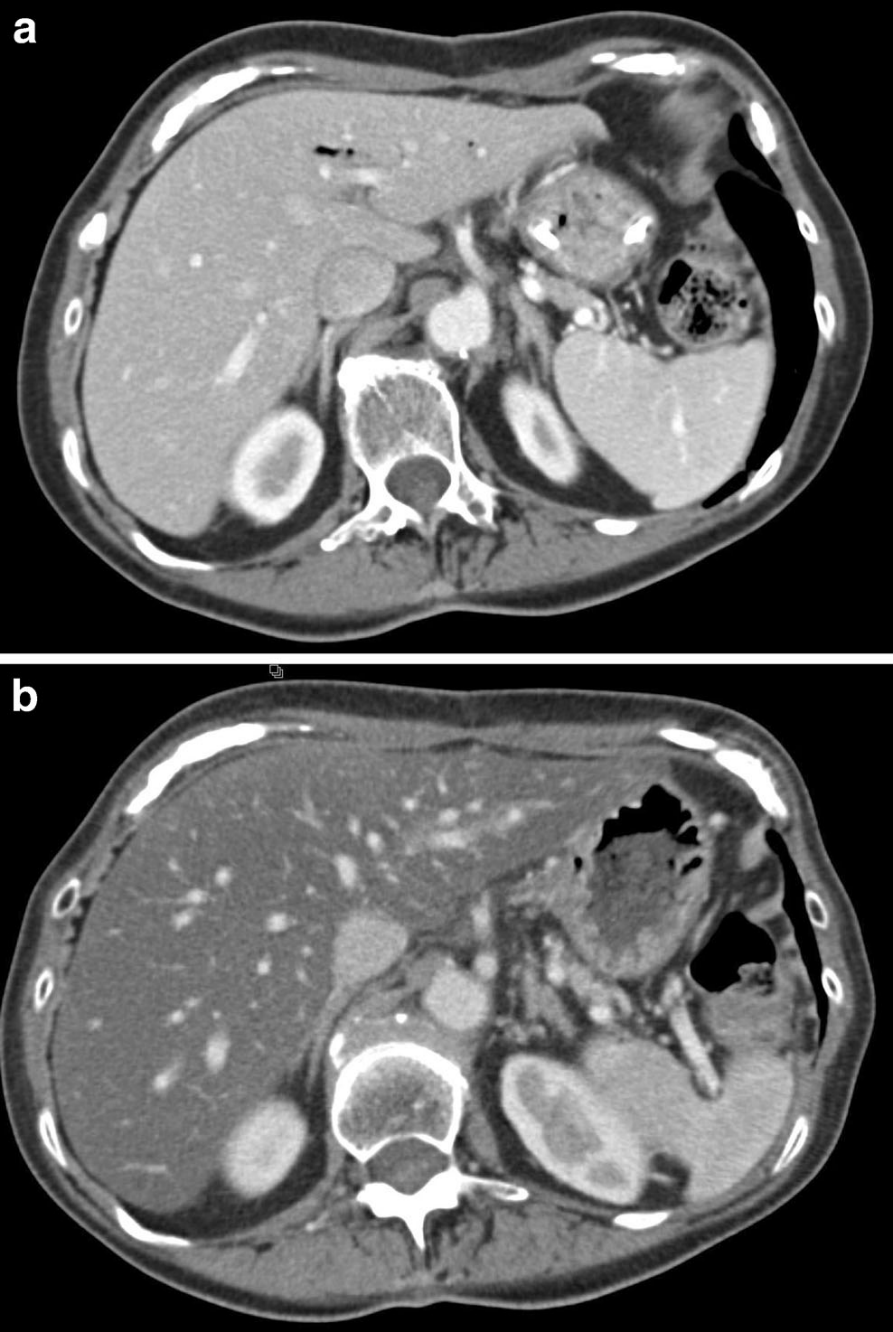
A 61-year-old male with colon cancer undergoing systemic treatment with oxaliplatin. a Axial contrast-enhanced CT pre-chemotherapy shows normal findings. b Axial CT status post 3 months of treatment with oxaliplatin shows development of diffuse fatty infiltration with hyperattenuating intrahepatic vessels consistent with steatosis
